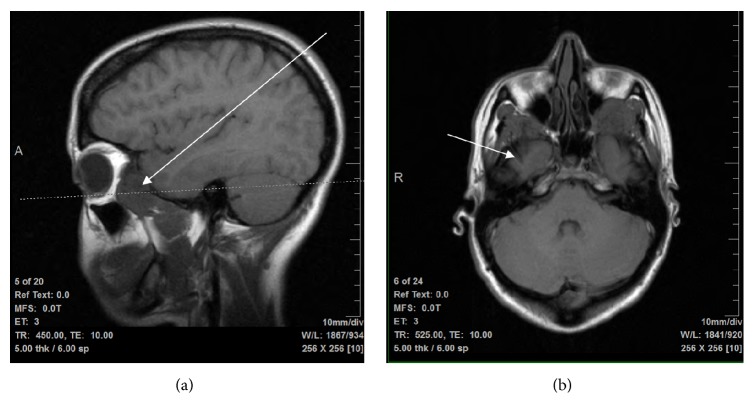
T1 MRI images of malignant PEComa metastasis exerting mass effect in both (a) sagittal and (b) axial views.
